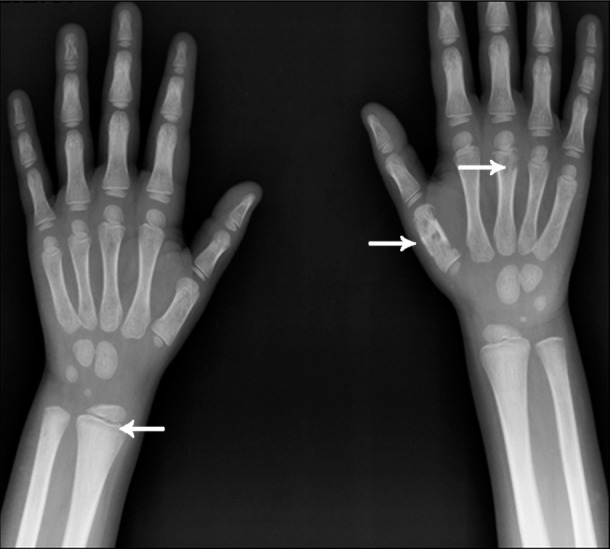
A 4-year-old girl with ALL presenting with pallor and wrist tenderness Wrist X-ray reveals metaphyseal lucent band (arrow head) in radial and ulnar metaphyses. Note the lucent bony lesions of the first and third metacarpal bone on the right side (arrows).
